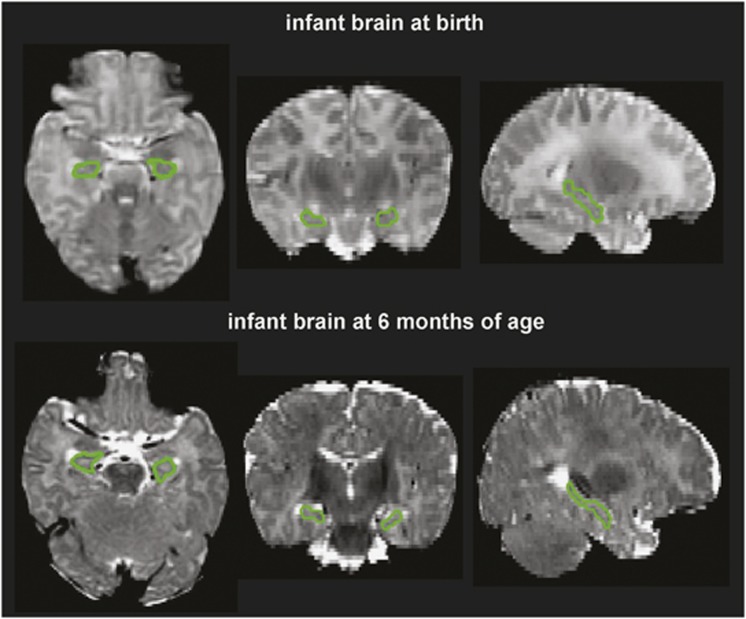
Segmented hippocampus from the brain of one infant at birth (top row) and 6 months (bottom row) of age. From left to right, the panels respectively show the axial, coronal and sagittal slices of the T2-weighted brain images.
